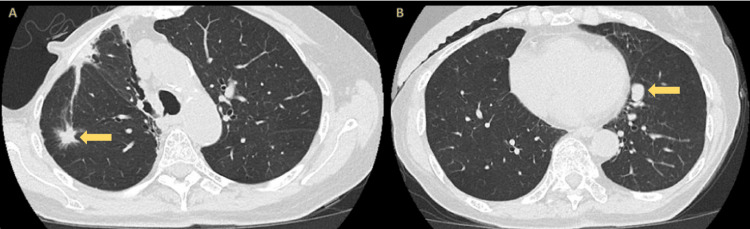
CT imaging demonstrating two suspicious pulmonary lesions Panel A: CT showing a spiculated lateral subpleural right upper lobe nodule measuring 11 × 12 mm (arrow), confirmed as moderately differentiated adenocarcinoma. Panel B: CT showing a juxta-cardiac left lower lobe nodule measuring 10 × 16 mm (arrow), confirmed as a typical carcinoid tumor. CT, computed tomography
